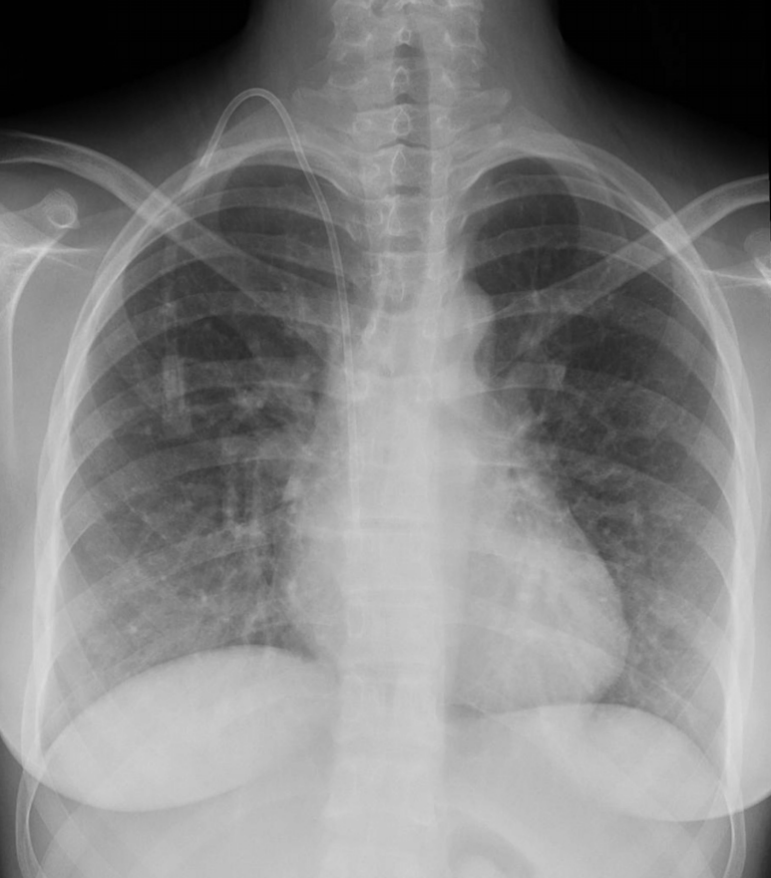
Chest antero-posterior X-ray showing a normal heart size, and a more marked pulmonary vasculature, particularly at basal level. A central venous catheter is evident as well.
